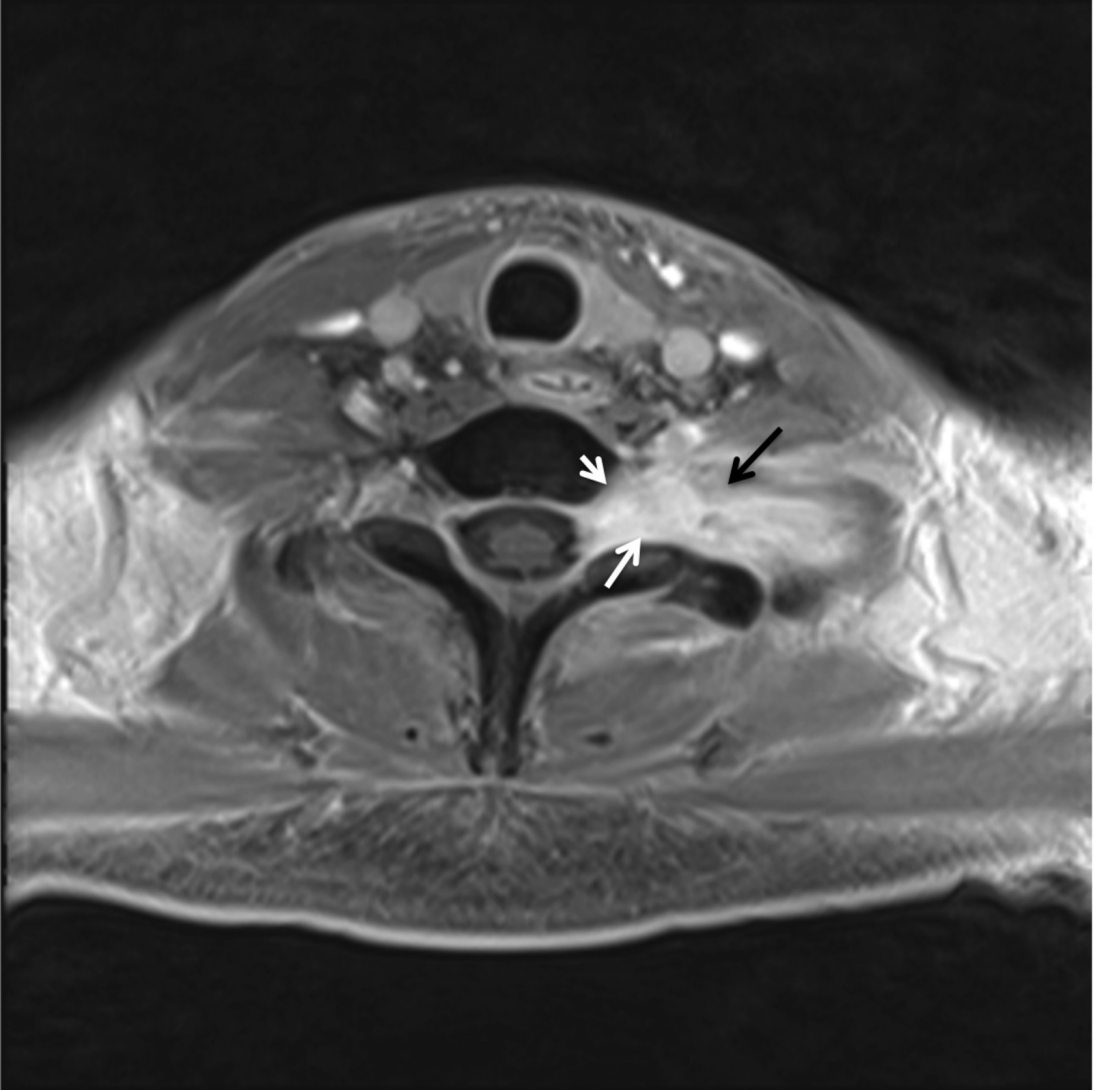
Axial magnetic resonance imaging (MRI) of patient 2. Representative axial MRI of patient 2 noting lesion of left cervical nerve root and adjacent soft tissues.
